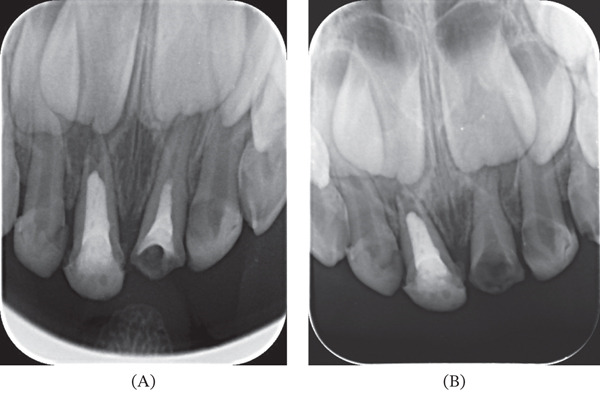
Initial periapical radiographs. (A) Initial radiograph and (B) canal disobliteration.
